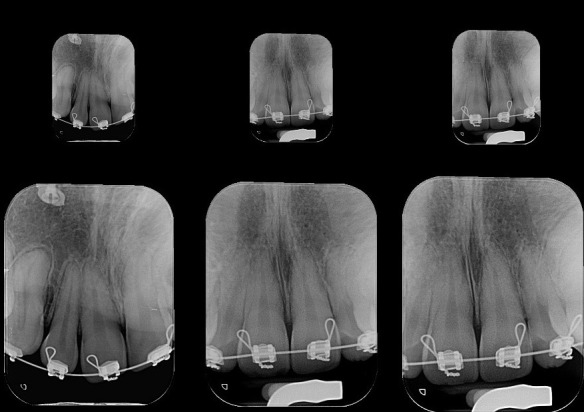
Post-surgery PSP digital periapical films.
